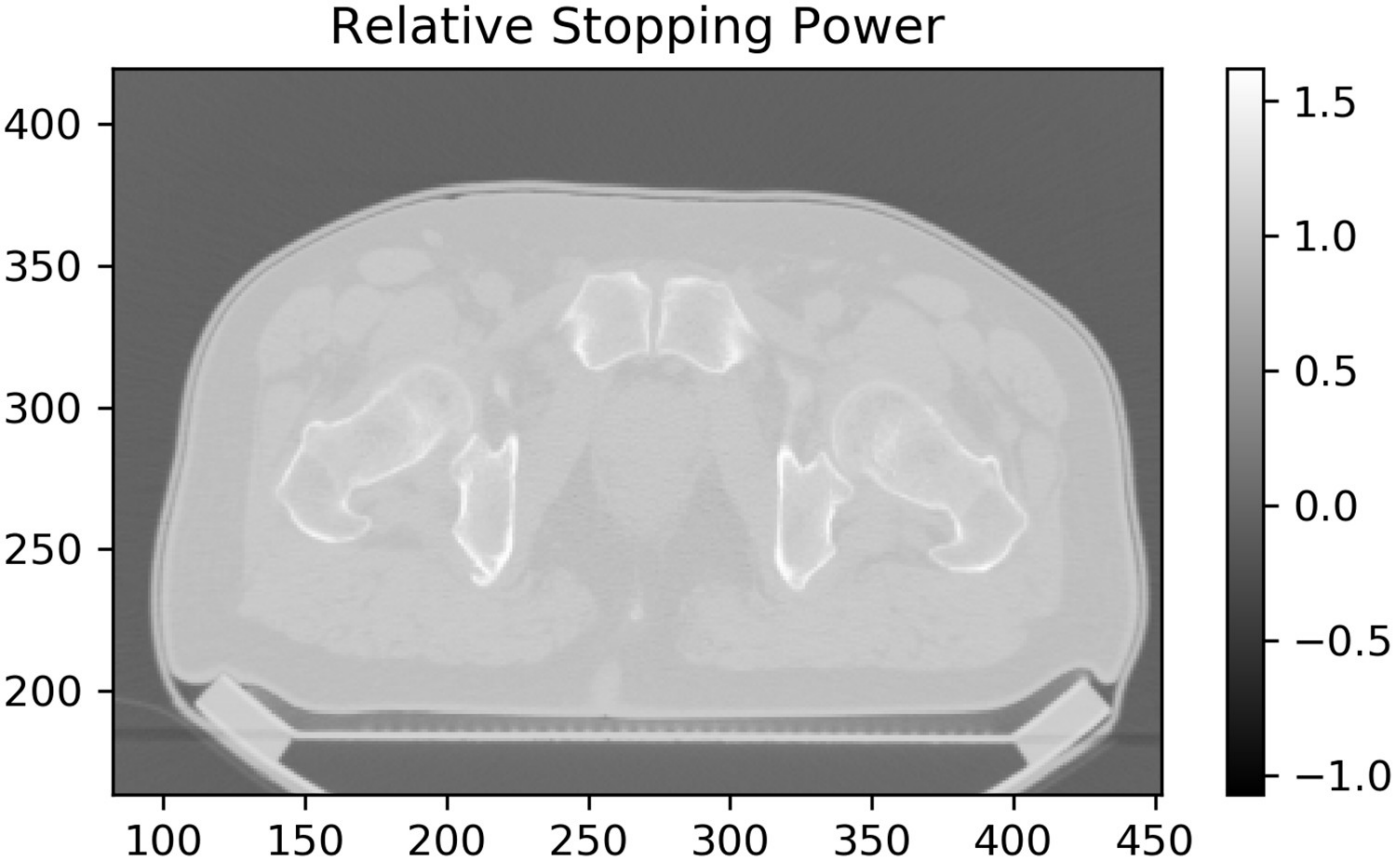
Tissue-to-water stopping power ratio for a typical pelvis CT image. Most of the body has relative stopping power similar to water, with exceptions being bone and hypodense fatty tissue. A thermoplastic shell holds the surface in the same position and bones are realigned to their planned position, so the largest change in stopping power comes from posterior prostate shifts into the anterior region of fatty tissue lateral to the rectum with SPR ~ 0.9.
